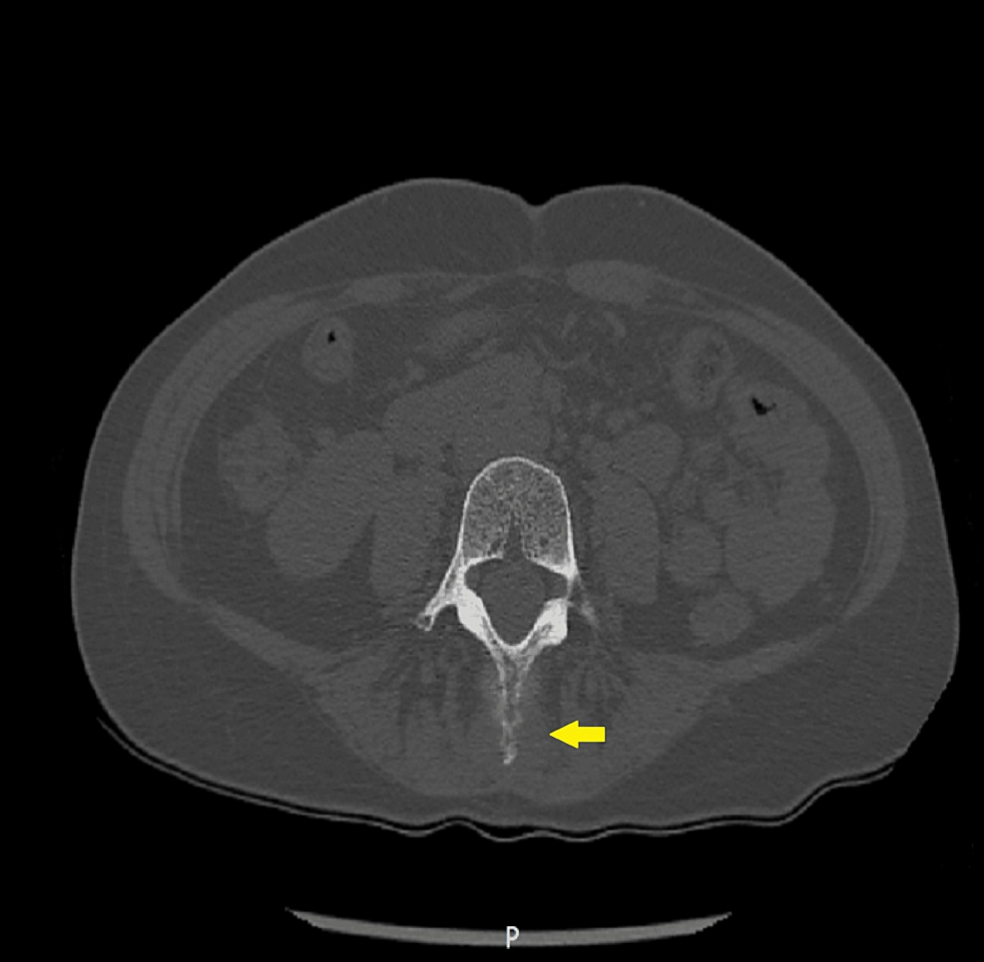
Abdominal CT-scan (spatial resolution: 512 × 512 and slice thickness: 1.25 mm). An osteolytic lesion on spinous process of lumbar fourth vertebra with soft tissue expansion CT scan with intravenous contrast substance – axial image.
